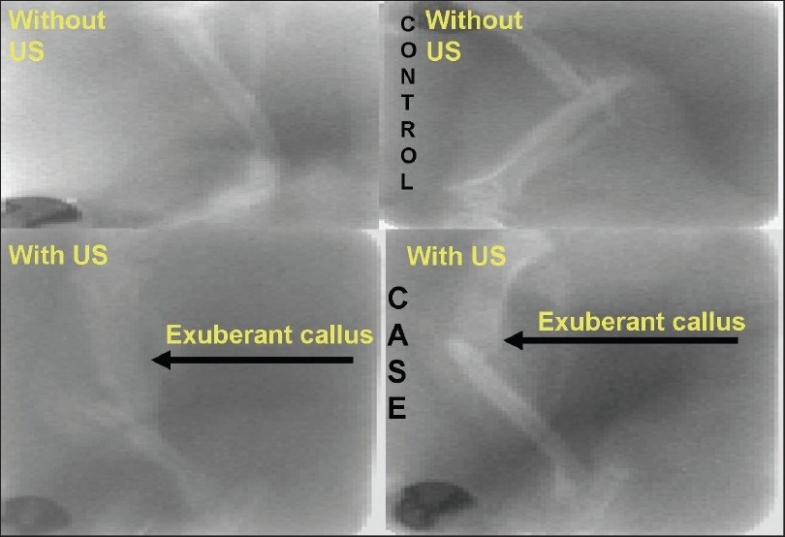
Difference in callus observed without ultrasound and with ultrasound
